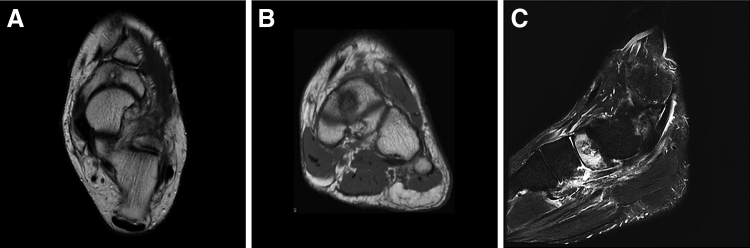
Preoperative magnetic resonance imaging of the left ankle. (A) T2 axial view, (B) T1 coronal view, and (C) sagittal T2 fast spin echo view. Images depict an osteochondral defect measuring 0.9 cm × 0.7 cm × 1.1 cm on the posterior facet of the navicular bone.
